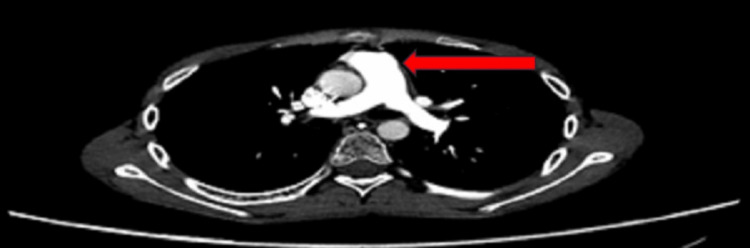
CT angiogram showing post-treatment disappearance of PE (arrow) CT: computed tomography; PE: pulmonary embolism
